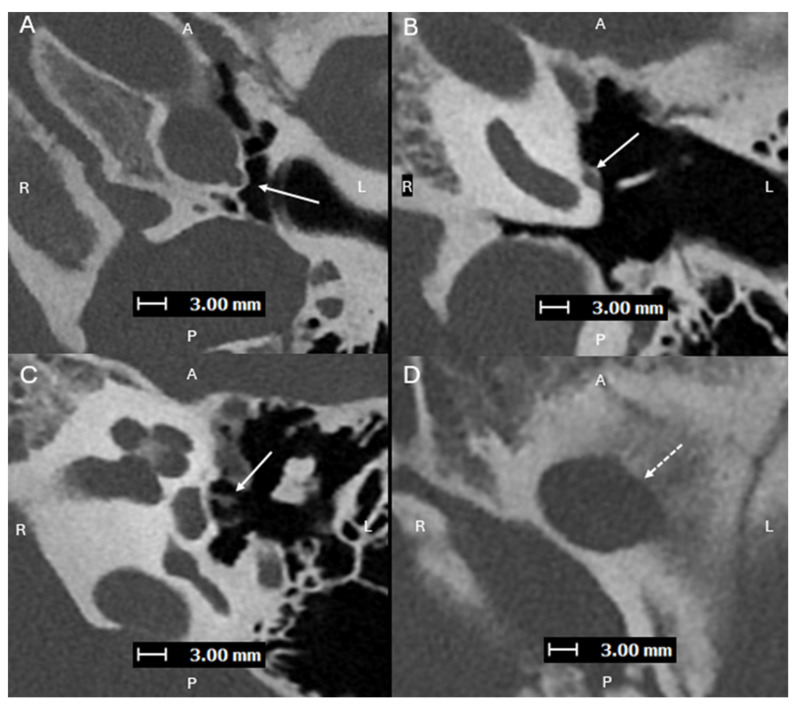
Axial oblique images (superior to inferior (A–C), with A, P, L, R for orientation donating anterior, posterior, left, and right respectively) from a PCD-CT temporal bone study—with same acquisition and reconstruction parameters as Figure 1—demonstrating a persistent L stapedial artery traveling inferiorly and passing through the obturator foramen of the stapes (C). (D) shows a normal foramen ovale (dashed line) with absence of the foramen spinosum, which is consistent with a persistent stapedial artery. Aberrant ICAs can be associated with a persistent stapedial artery (PSA), which is an abnormal vessel that arises from the petrous ICA and passes through the obturator foramen of the stapes [10]. The posterior division of the upper stapedial artery branch becomes the middle meningeal artery (MMA). With the normal embryologic atrophy, the distal portion of the MMA is supplied by the internal maxillary artery through the foramen spinosum. If this fails to happen, the territory of the middle meningeal artery will be supplied by the collateral connection with the ophthalmic artery or by a PSA, and absence of the foramen spinosum is expected [11]. A PSA should be recognized prior to stapes surgery. The improvement of spatial resolution with photon counting detector (PCD)-CT has been proven to provide higher performance in detection of sub-centimeter vascular lesions compared to conventional energy-integrated detector (EID)-CT [12].
